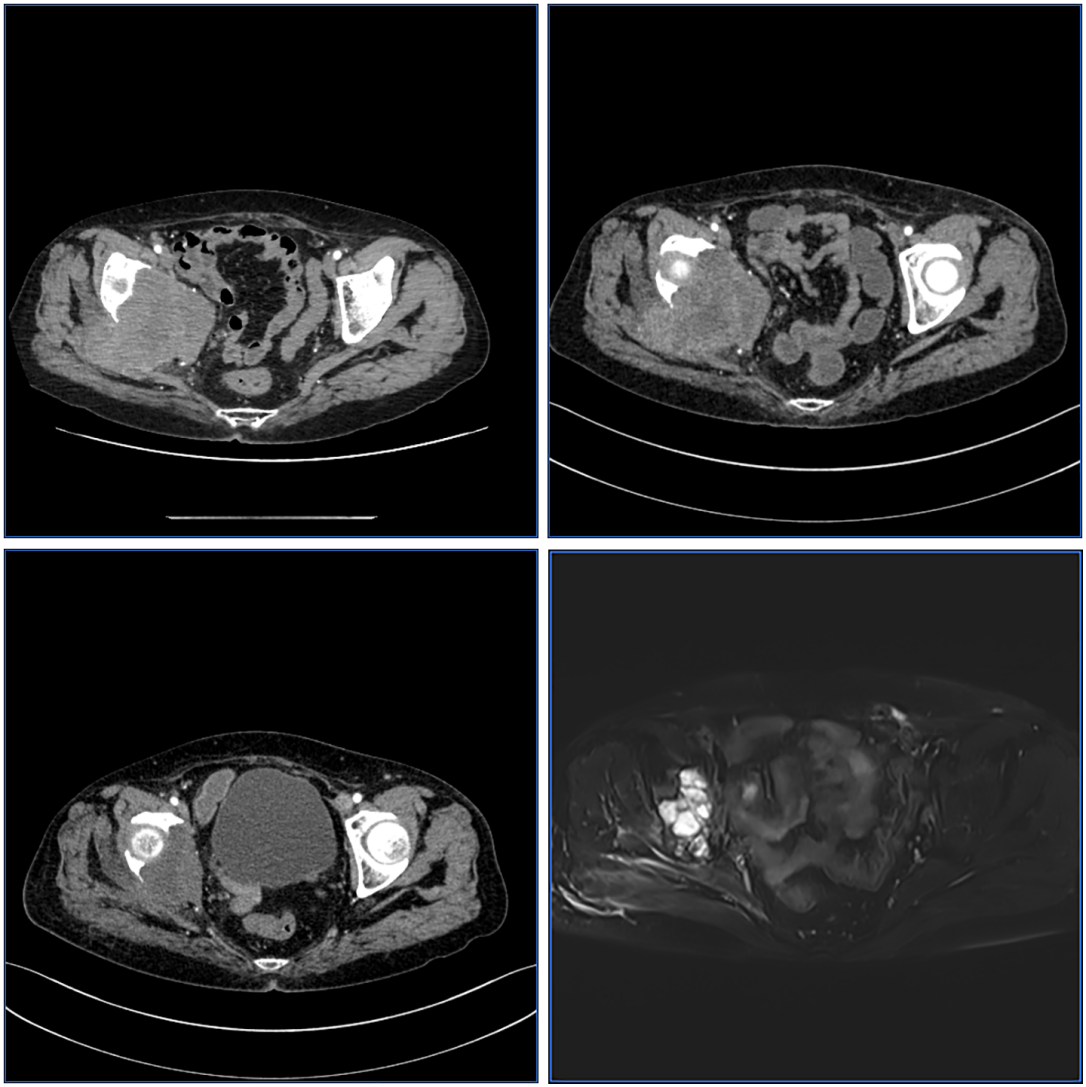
CT/MRI scans showing pelvic cross-sections at different treatment stages: “Before SCART,” “1 month after SCART,” “3 months after Lattice,” and “6 months after RT.” Each panel displays changes in tumor size and tissue density over time.
